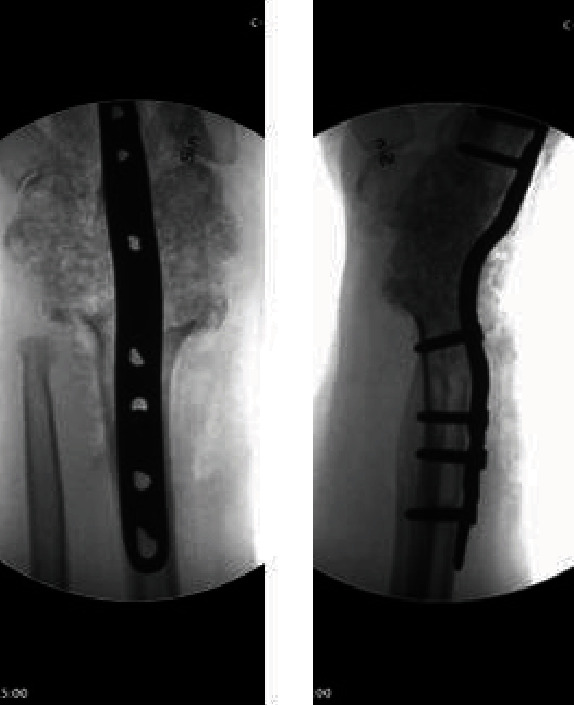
Intraoperative radiographs after bone grafting and dorsal plate fixation.
